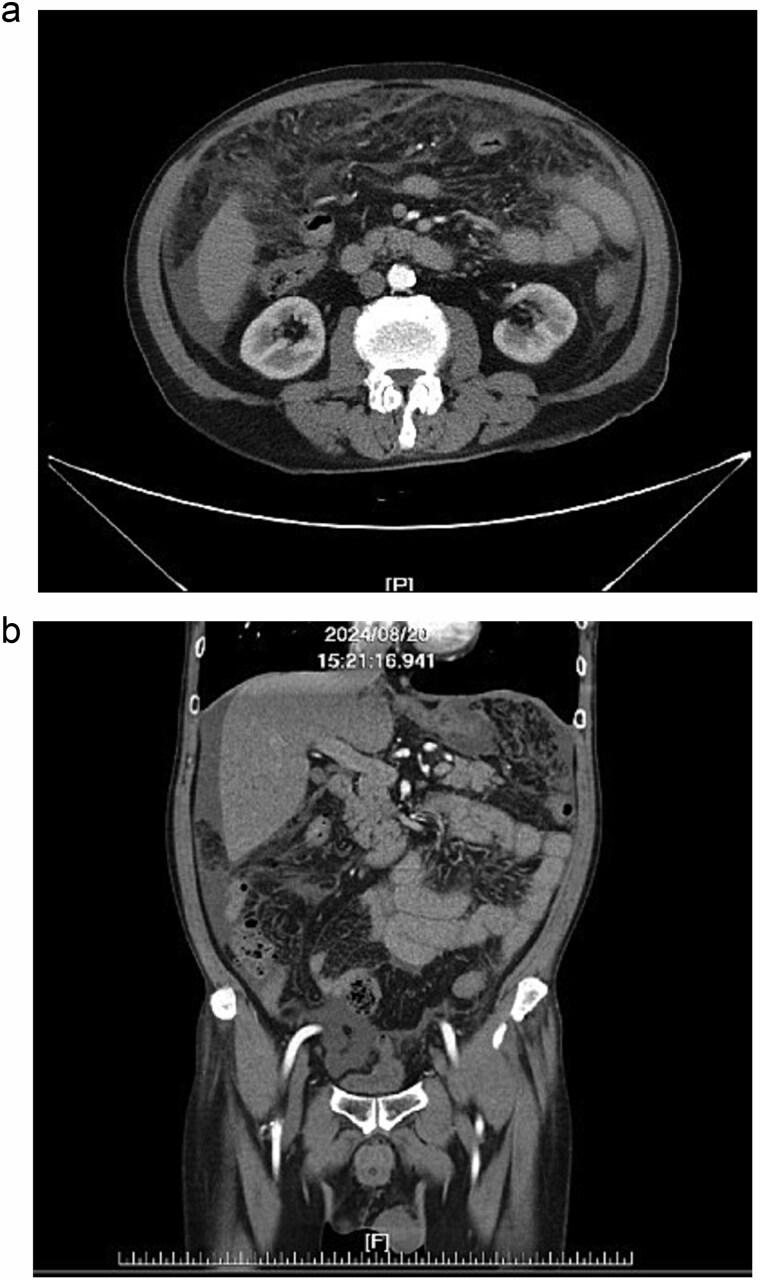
(a, b) Abdominal CT, peritoneal thickening with multiple miliary nodules and omental cake with ascites.
